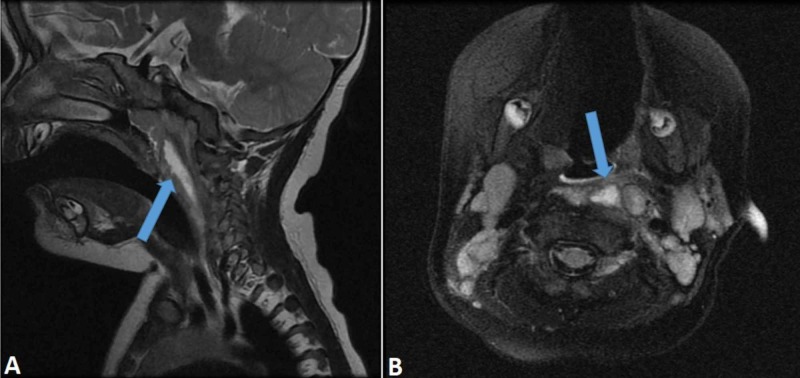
MRI of the neck confirming the presence of an abscess (arrow). (A) Sagittal view. (B) Axial view. MRI, magnetic resonance imaging
